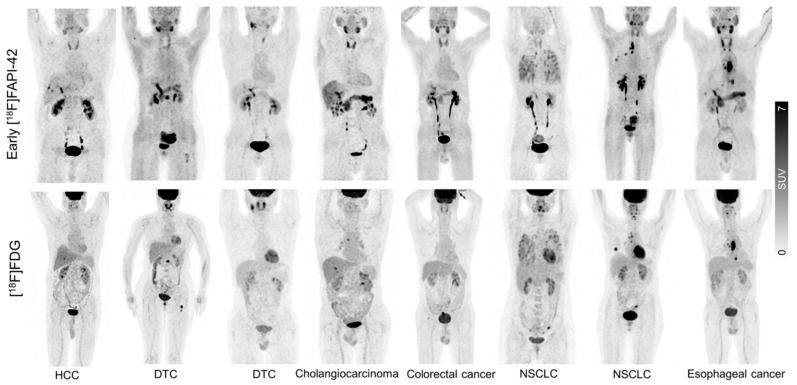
Example of maximum-intensity projection images of early [^18^F]AlF-FAPI-42 (mean = 22 min) and [^18^F]FDG PET/CT in patients with different types of cancer. HCC = hepatocellular carcinoma; DTC = differentiated thyroid carcinoma; NSCLC = non-small cell lung cancer. Images originally published by Mu et al. [191].
